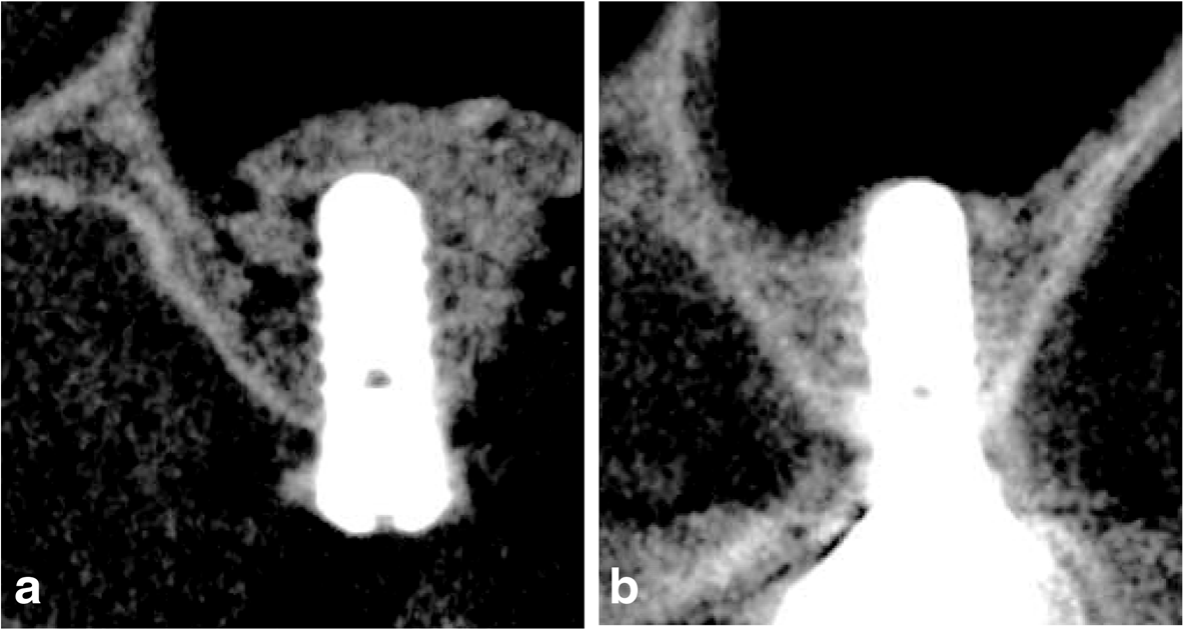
Radiographic examination: The relationship between changes in the maxillary sinus floor associated with a reduction in the grafted bone and the implant tip (a immediately after surgery, b 5 years after surgery)
